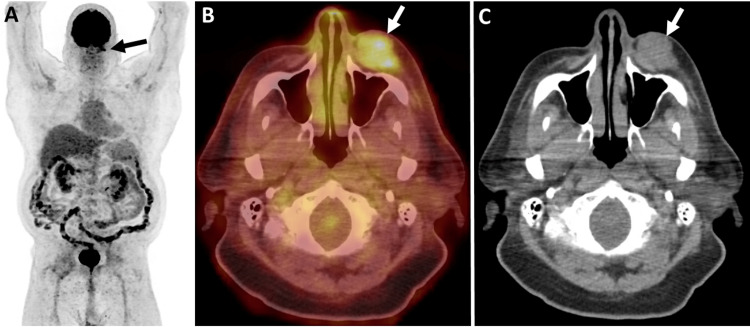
Initial staging 18F-FDG PET/CT: (A) maximum intensity projection, (B) trans-axial fused PET/CT, and (C) unenhanced axial CT images demonstrating focal increase FDG uptake in the left face (black arrow in A) corresponding to the metabolically active skin and subcutaneous soft tissue lesion anterior to the left maxillary sinus without invasion in the left maxillary sinus (white arrow in B and C). There is no other abnormal metabolic active lesion in the whole body (A). 18F-FDG PET/CT: 18F-fluorodeoxyglucose positron emission tomography/computed tomography
